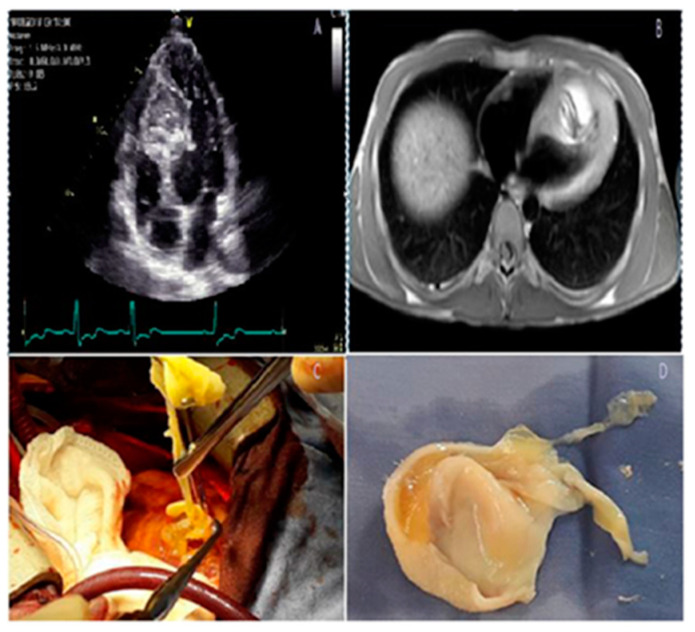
A large cardiac hydatid cyst in the interventricular septum. (A) Echocardiographic frame in an apical 4-chamber view showing a large cystic mass splitting the interventricular septum (IVS). (B) Magnetic resonance image showing hydatid cyst located in the IVS. (C) Cystectomy and membrane extraction. (D) Germinative membrane. Fennira S, et al. Int. J. Infect Dis. 2019;78:31–33 [74].
